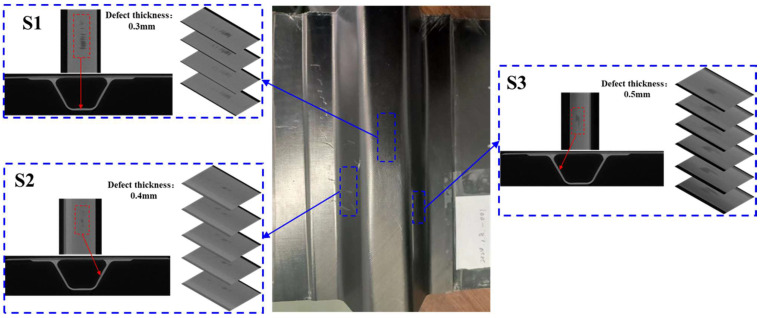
CT damage images of CFRP stringer.
